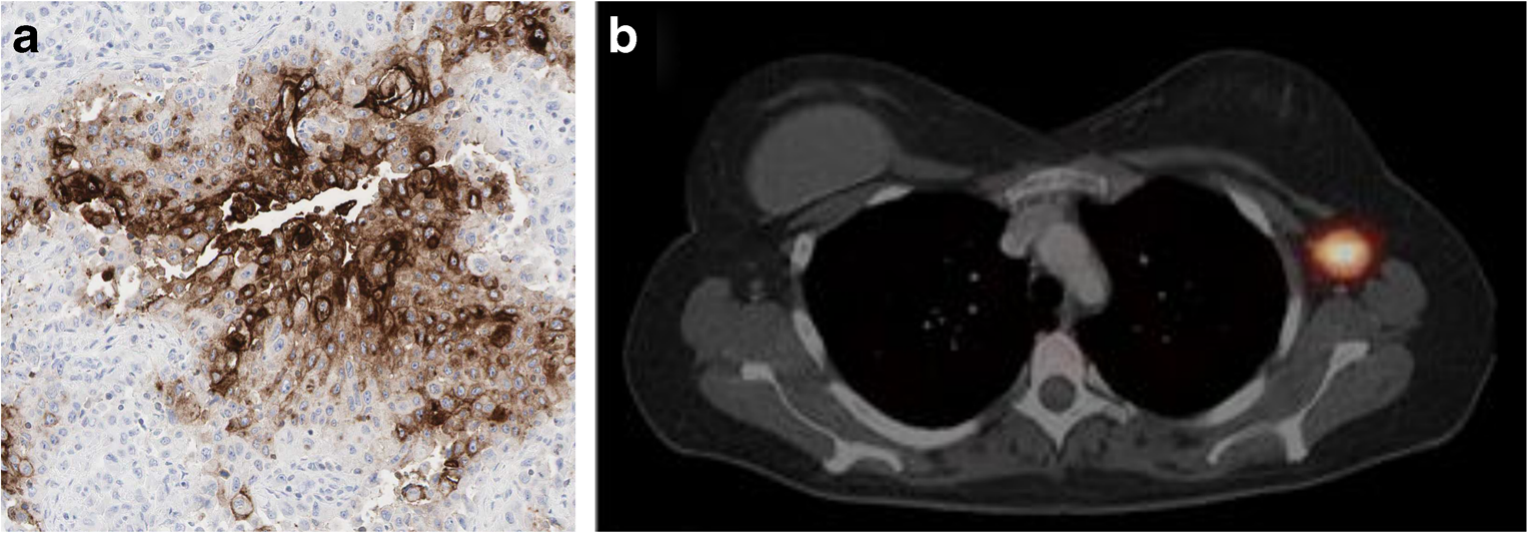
a CEA expression in human PDAC tissue (protein atlas [56]) b SPECT/CT image in a 38-year-old patient acquired 24 h after injection of ^111^In-IMP288 (185 MBq, 25 μg) pretargeted with 75 mg of TF2 (1-day interval) [80]
